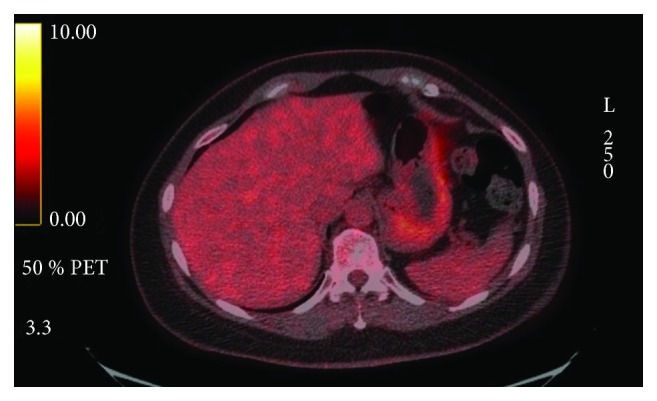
PET/CT after 4 cycles of Pembrolizumab.
